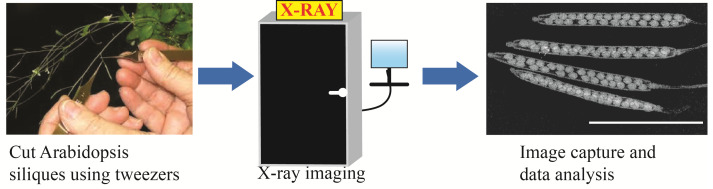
Arabidopsis* siliques using the Inspex 20i X-ray machine
